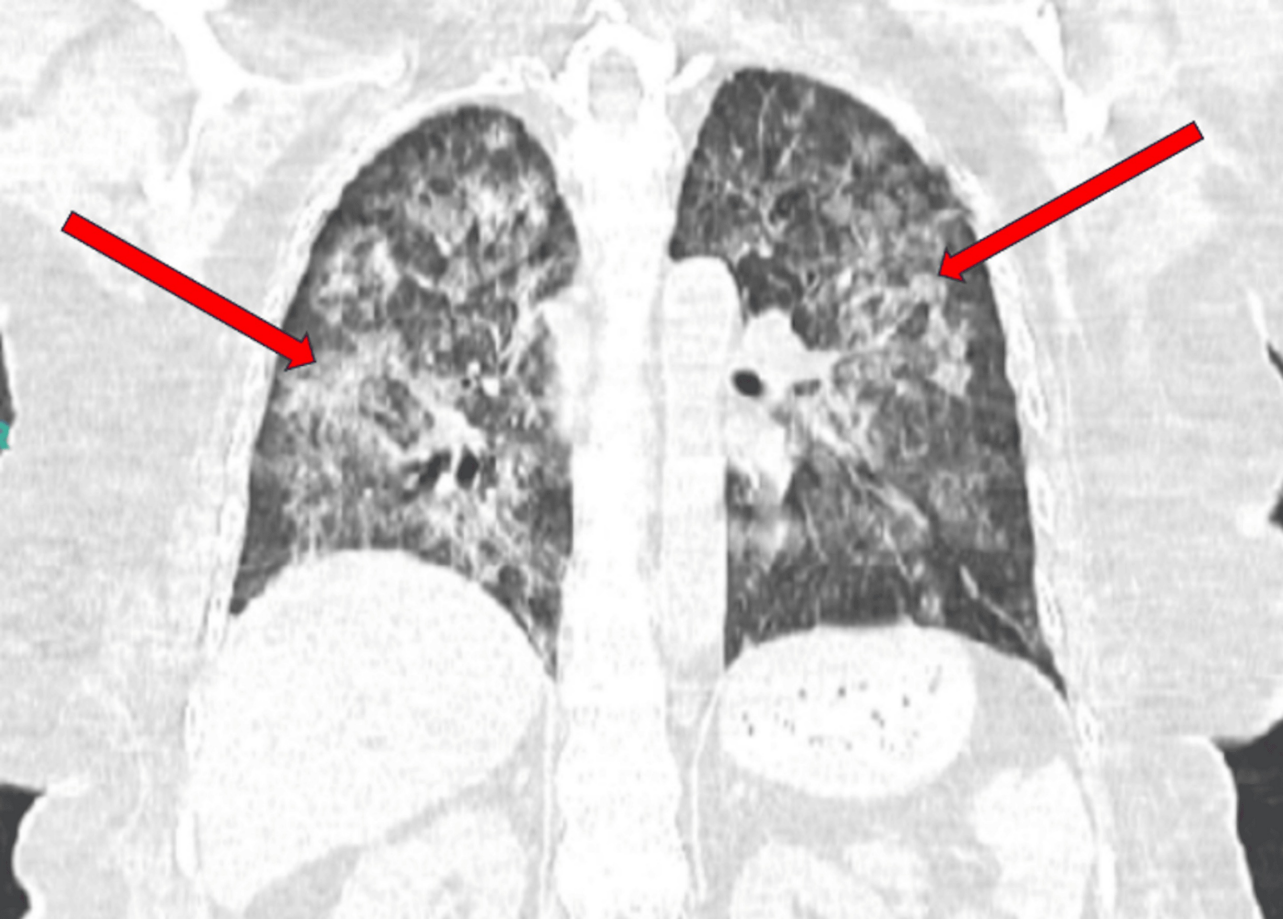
Coronal chest CT in lung window demonstrating diffuse, bilateral ground-glass opacities with patchy areas of consolidation throughout both lungs. Given the bronchoscopy findings of DAH, the CT findings represent bloody alveolar infiltrates. Red arrows indicating areas of ground-glass opacities. CT: Computed tomography, DAH: Diffuse alveolar hemorrhage
